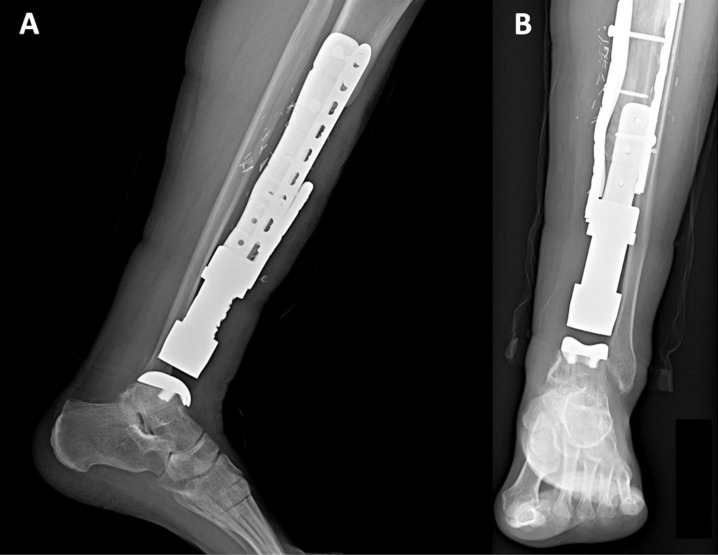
X-rays of the Case 2’s distal tibia and ankle megaprosthesis in a (A) lateral and (B) anteroposterior view.
